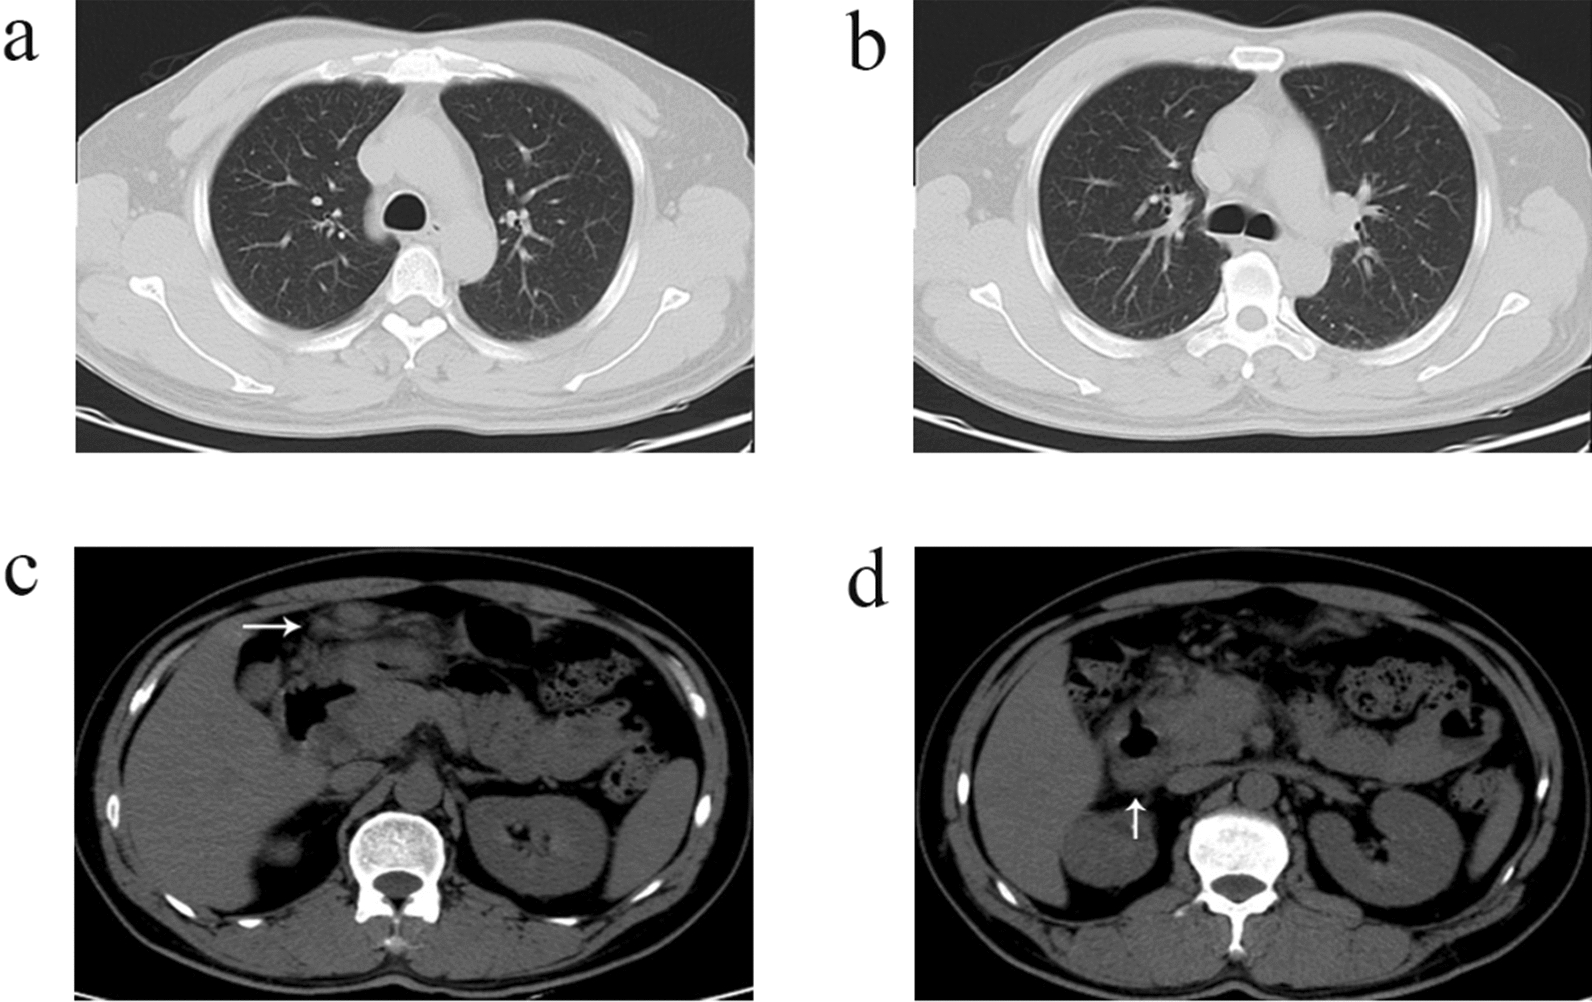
Images of chest CT and abdominal CT scan. Images a, b of chest CT showing no obvious primary tuberculosis foci in the lungs. The CT image c of the abdomen showing exudation and multiple enlarged lymph nodes in the antrum and duodenum. Image d of the abdomen CT showing uneven thickening of gastric antrum and duodenal mucosa
